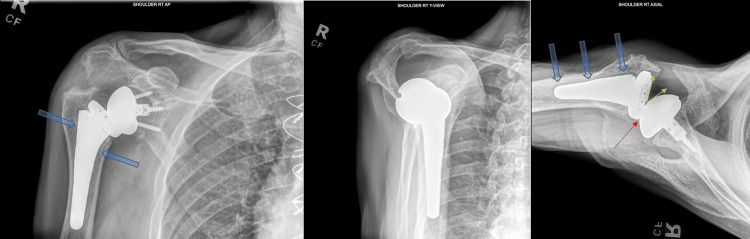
Preoperative radiographs of the right shoulder The images show anterior humeral subluxation (green arrowed v-sign demonstrating increased space at the prosthesis articulation anteriorly with loss of concentric alignment of the humeral component on the glenosphere); posterior metal-on-metal component contact of the humeral and glenoid components (red thin arrow); and slight lucency around the humeral component (blue thick arrows)
